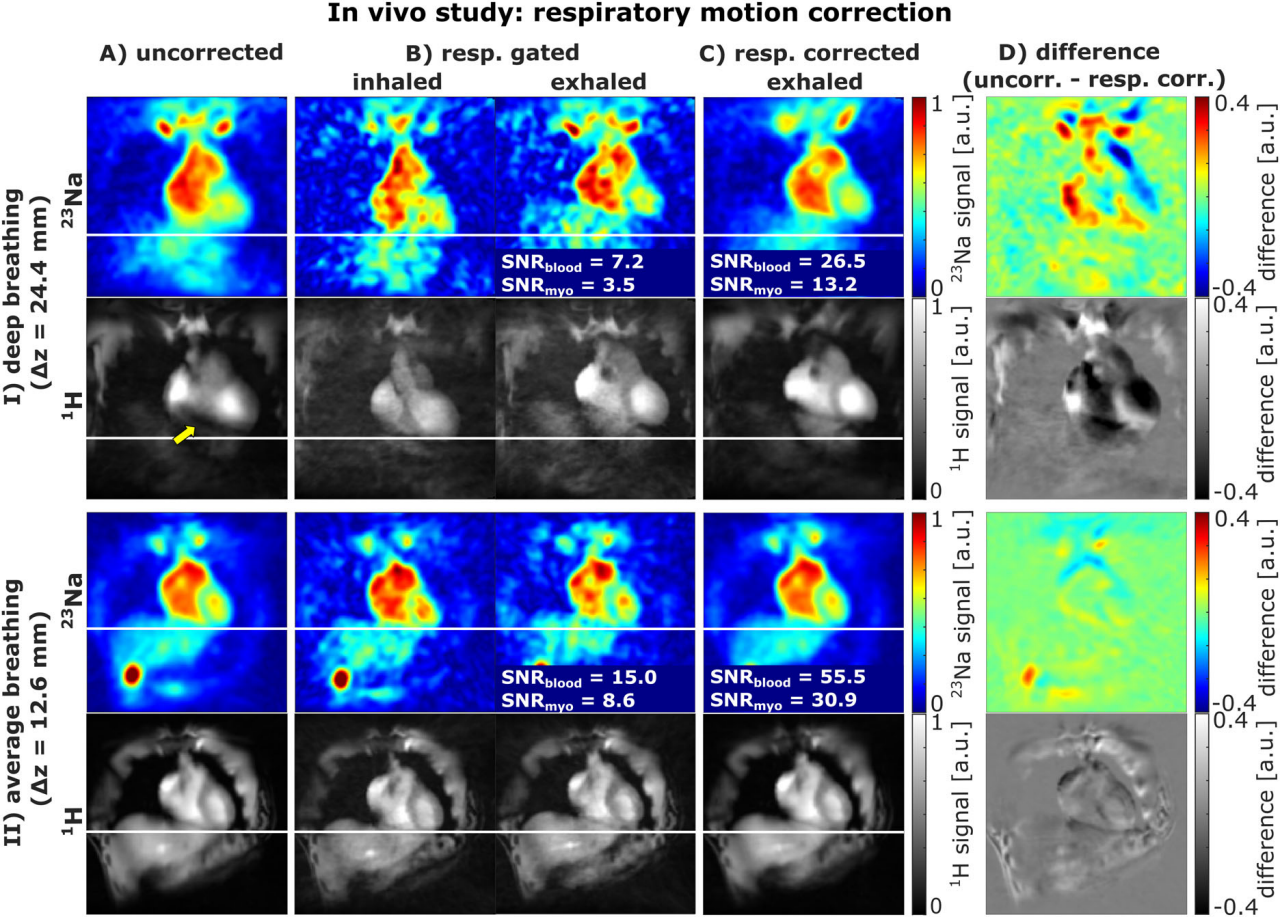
Influence of respiratory motion gating and correction on ^23^Na and ^1^H images for the in vivo measurements of two subjects with different breathing depth. For the subject with deep breathing (I), the uncorrected image (A) exhibits severe motion blurring and even signal dropouts in the ^1^H MRI (yellow arrow). With a more average breathing depth (II), these artifacts are less pronounced. Respiratory‐gated reconstruction (B) reduces motion‐induced blurring in both subjects and visualizes the respiratory displacement between the fully‐inhaled and fully‐exhaled bins. Applying respiratory motion correction (C) diminishes motion‐related errors while preserving higher SNR than respiratory gating. The quantitative impact of the respiratory motion correction is evident in the difference maps (D) and is particularly pronounced for the subject with deep breathing (I).
